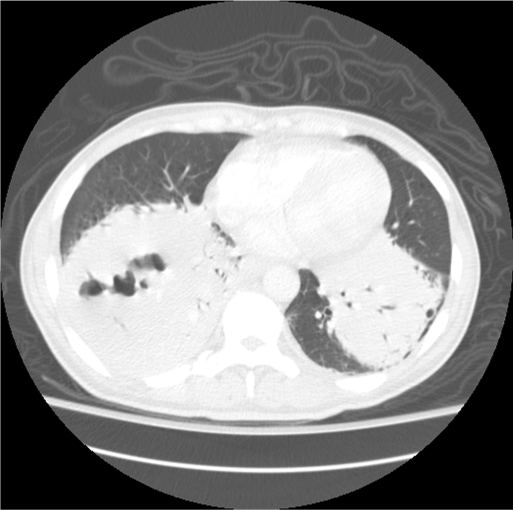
Subsequent CT scan performed in April 2008. CT of thorax showing cavitation of right lower lobe previously low-grade BALT lymphoma.
